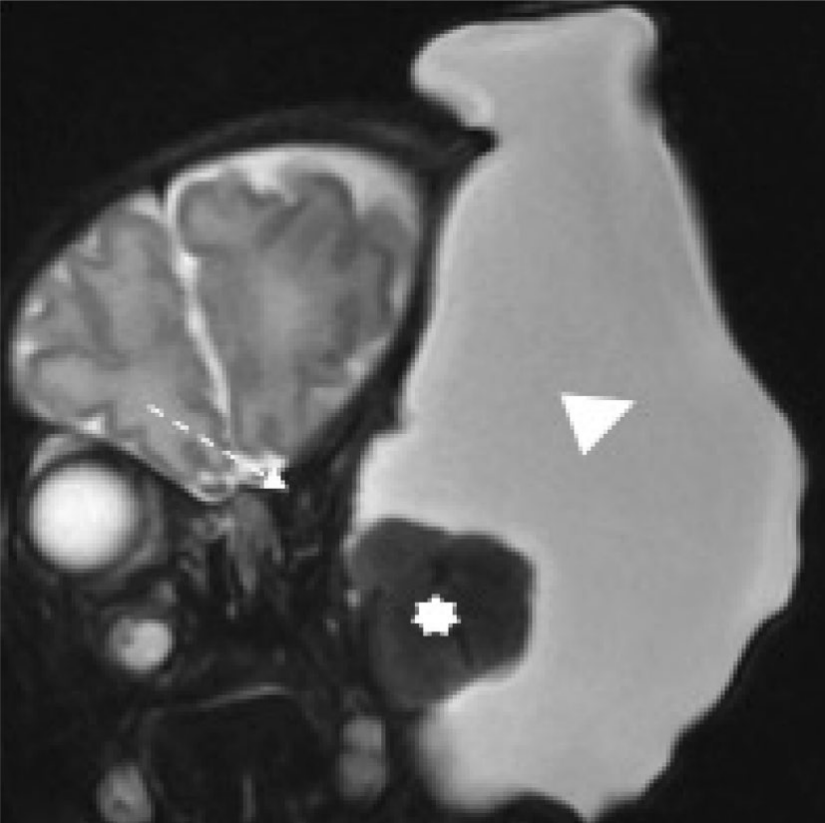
Postnatal MRI of the head and neck. Coronal T2-weighted MRI of the head and neck on day 1 of life again demonstrates the large left-sided cystic mass (arrowhead) with a solid component medially (star). There is distortion and displacement of adjacent structures including the left orbit (dashed arrow). Some decrease in the size of the cystic component is noted due to drainage a few hours previously postnatally
